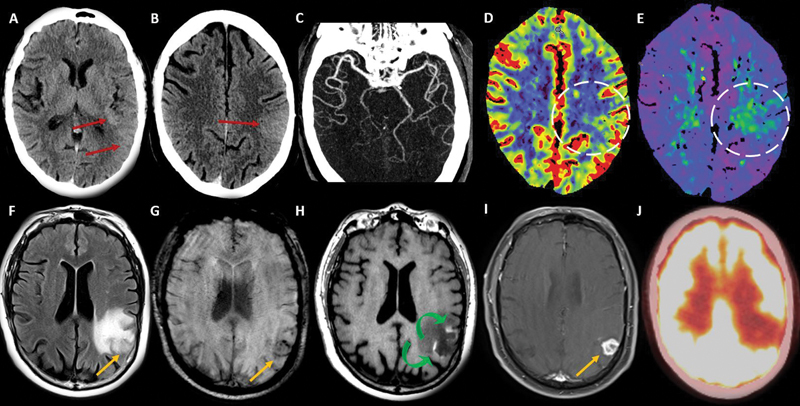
Head computed tomography (CT) stroke protocol (
